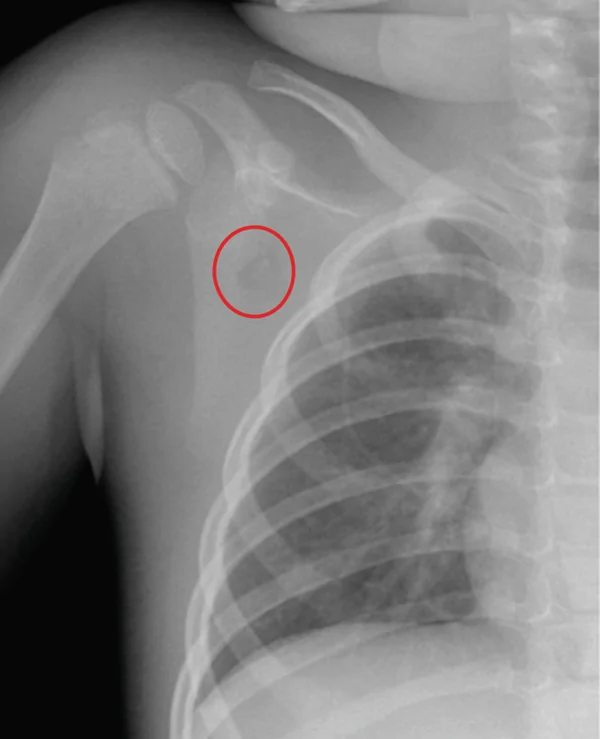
Section of anteroposterior radiograph of the chest demonstrating location of corticotomy (red circle).
